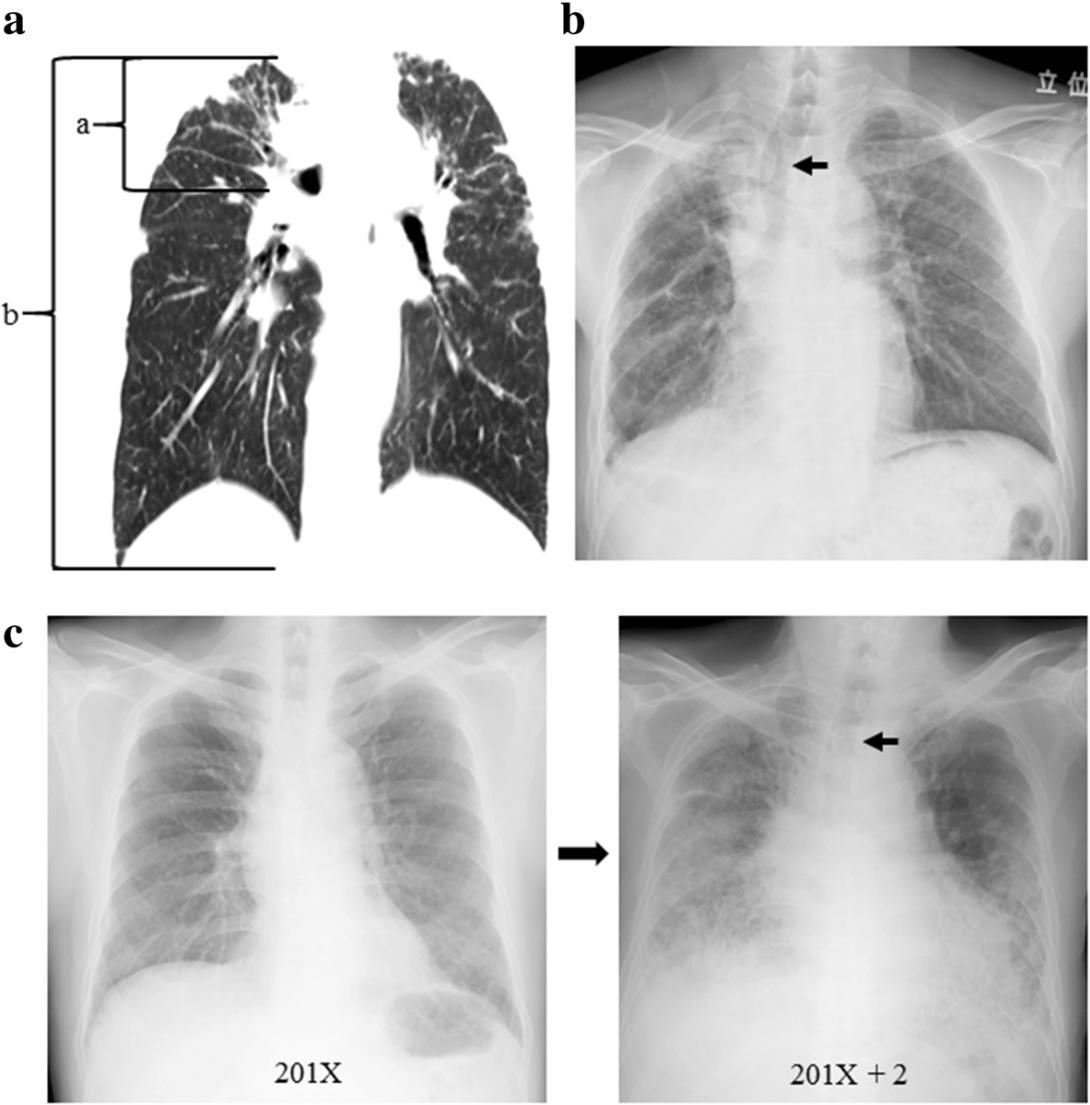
Coronal section image of chest computed tomography (CT) of a patient with PPFE (a). The ratio of the length of the lung apex to the hilum (a) and the length of the apex to the base (b) of the right lung was measured using axial section images on chest CT. Representative chest radiographs of rightward tracheal deviation (arrows) in a PPFE patient (b) and an IPF patient with the evolution of disease (c)
